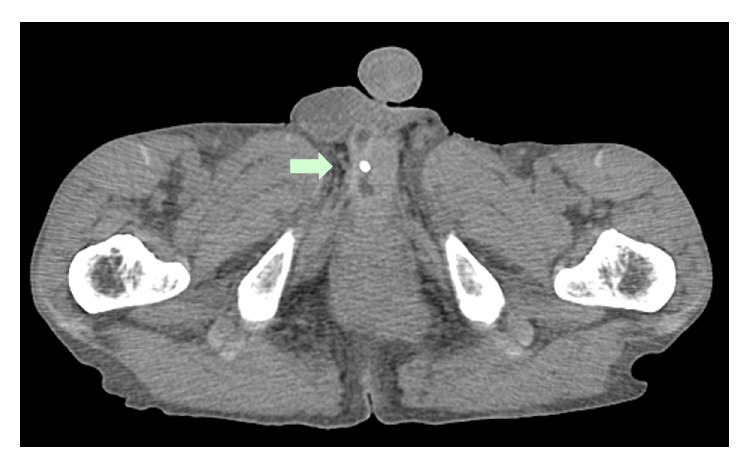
Calculus in the pendulous urethra on plain abdominal CT (arrow).
